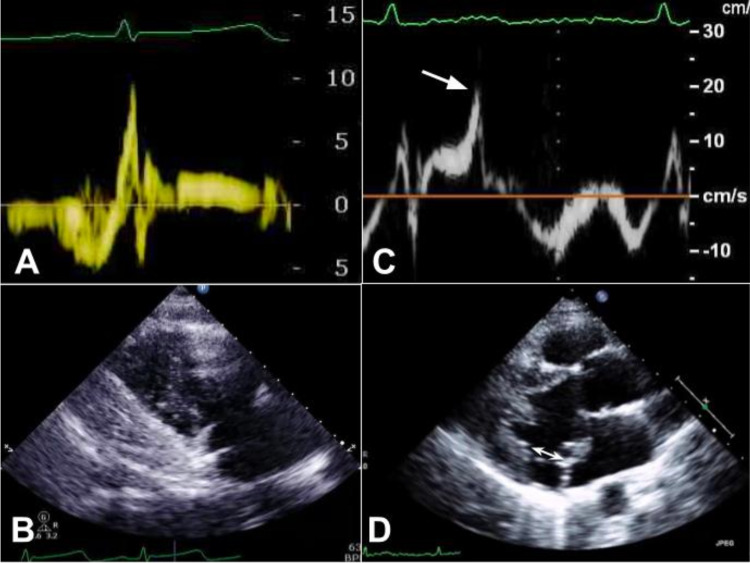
Initial echocardiogram (A, B) and follow up echocardiogram (C, D) A. Tissue Doppler of the mitral annulus without mid-systolic spike. B. Parasternal long axis view, no mitral annular disjunction appreciated. C. Tissue Doppler of the mitral annulus with prominent mid-systolic spike of 20 cm/s - Pickelhaube sign (arrow). D. Parasternal long axis view with significant mitral annular disjunction (double arrow) of 19 mm.
